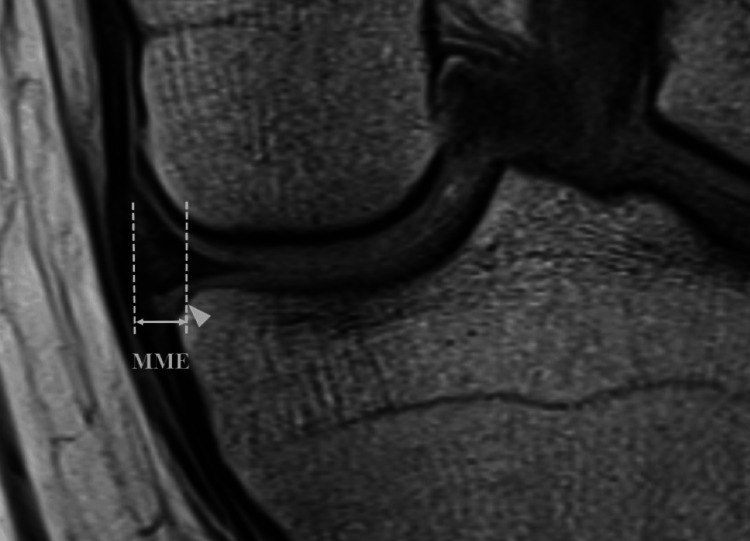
Measurement of MME using MRI The MME was determined by measuring the distance from the innermost edge of the MM to the medial border of the tibia, excluding the osteophyte, in the coronal slice where the intercondylar ridge was the greatest. The arrowhead indicates the medial border of the tibia, excluding the osteophyte. It marks the location of the inflection point where the horizontal line of the joint surface drops into a curved depression. MME: medial meniscal extrusion, MRI: magnetic resonance imaging, MM: medial meniscus
